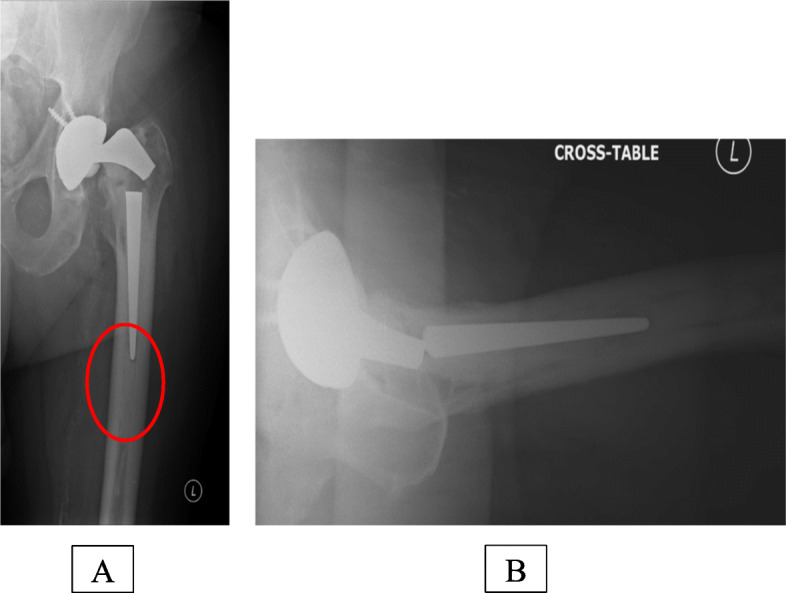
a, b Radiograph showing broken femoral stem. Note the cement column beyond the tip of the femoral stem in figure a
